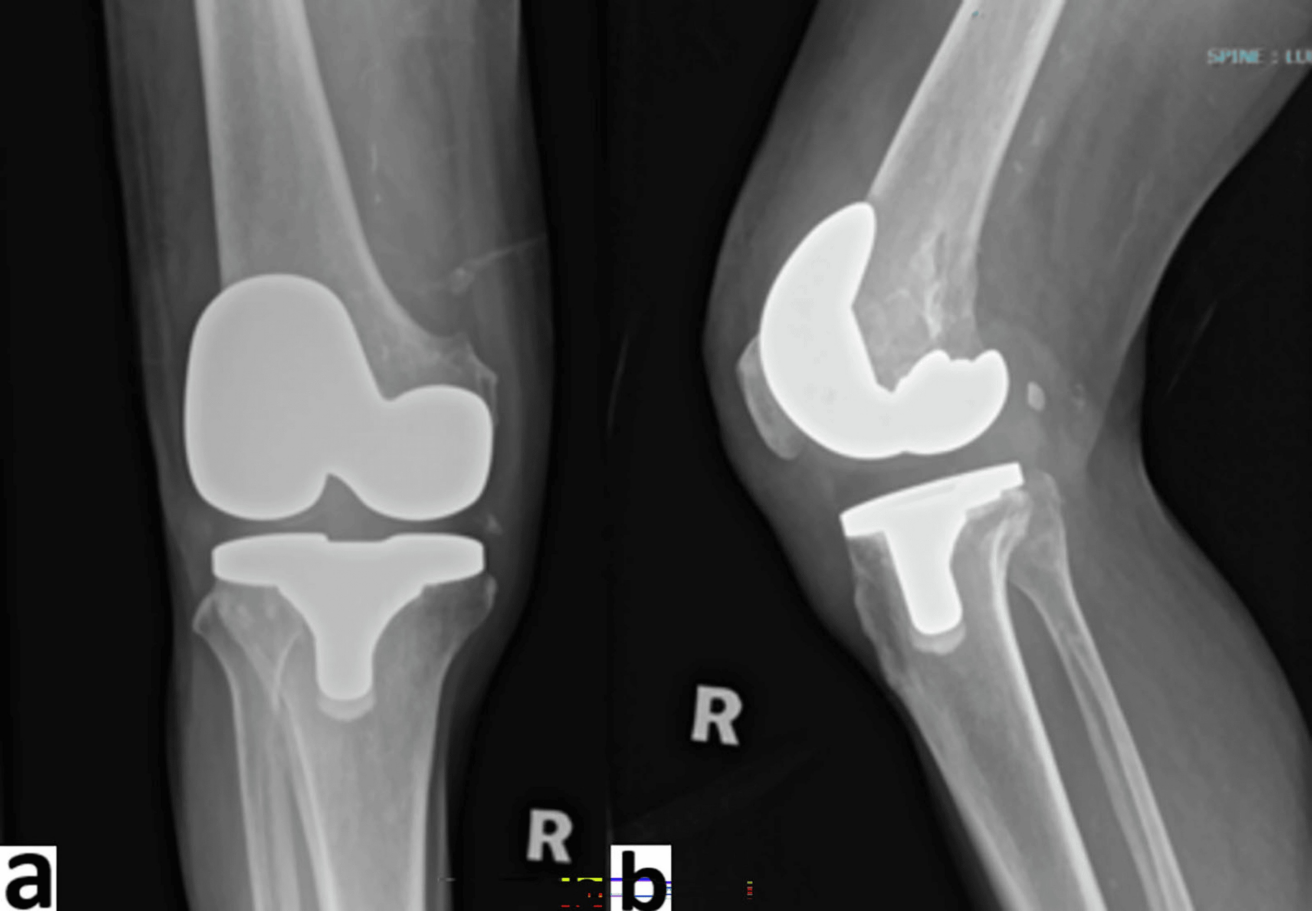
Radiographs of right knee a) anteroposterior view; b) lateral view
